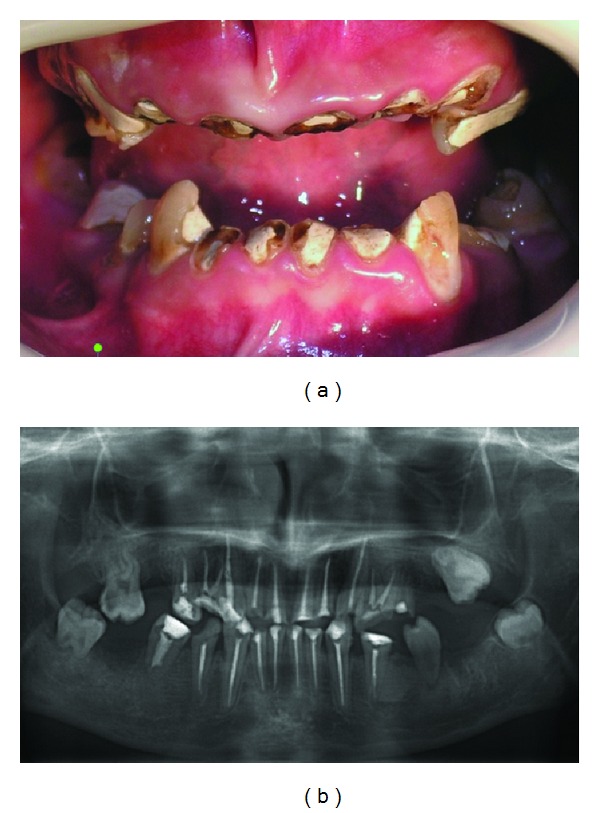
Intraoral (a) and radiographic (b) view of the patient after initial periodontal treatment and endodontic treatments.
